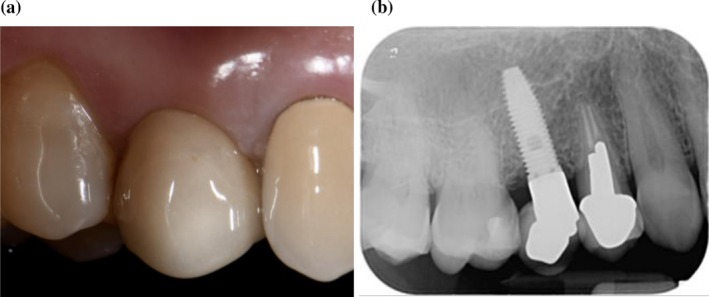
Clinical (a) and radiographic (b) appearance of test implant in regio 1.5 at T0.
